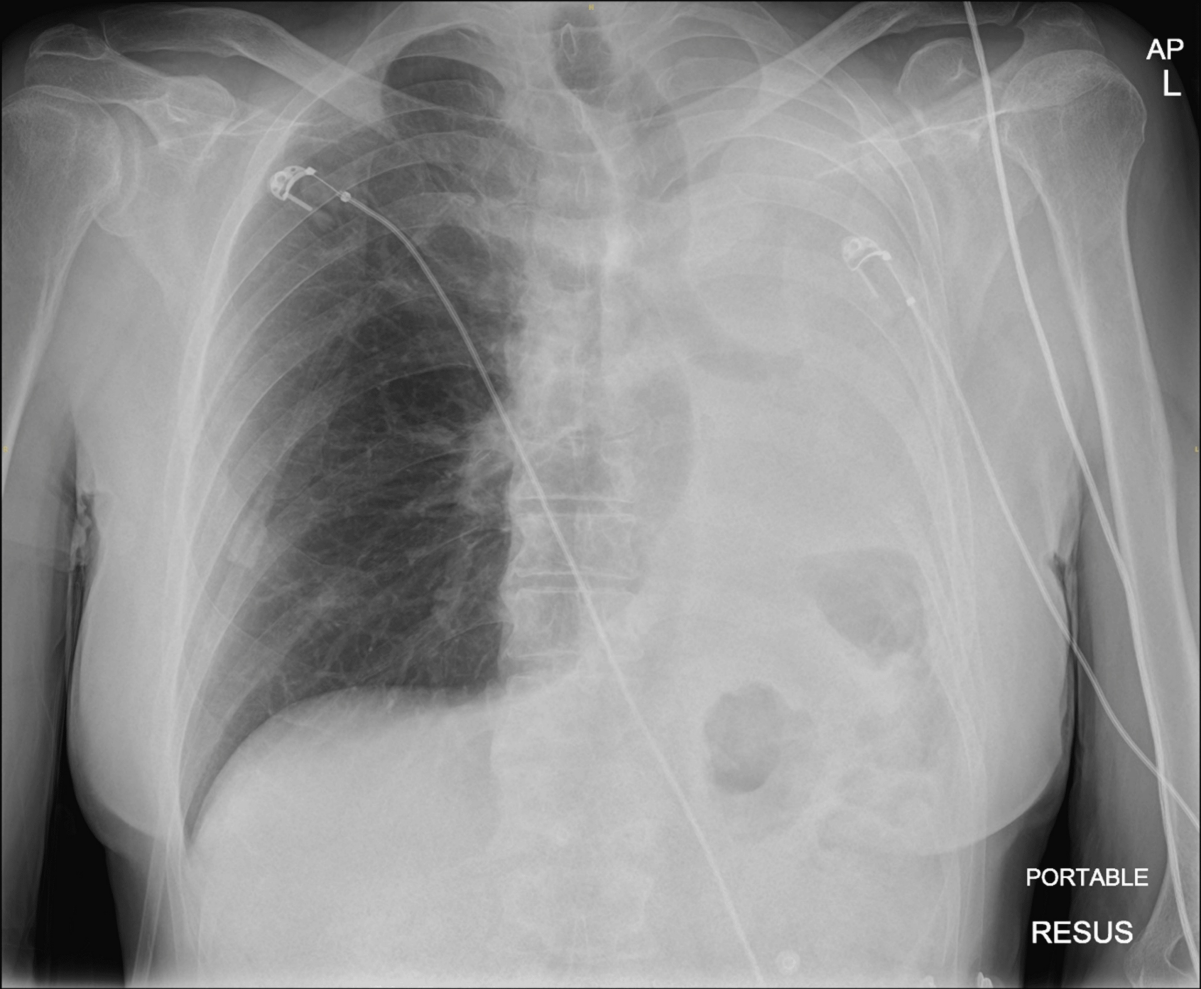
Chest X-ray showing complete white-out of the left lung with tracheal deviation.
